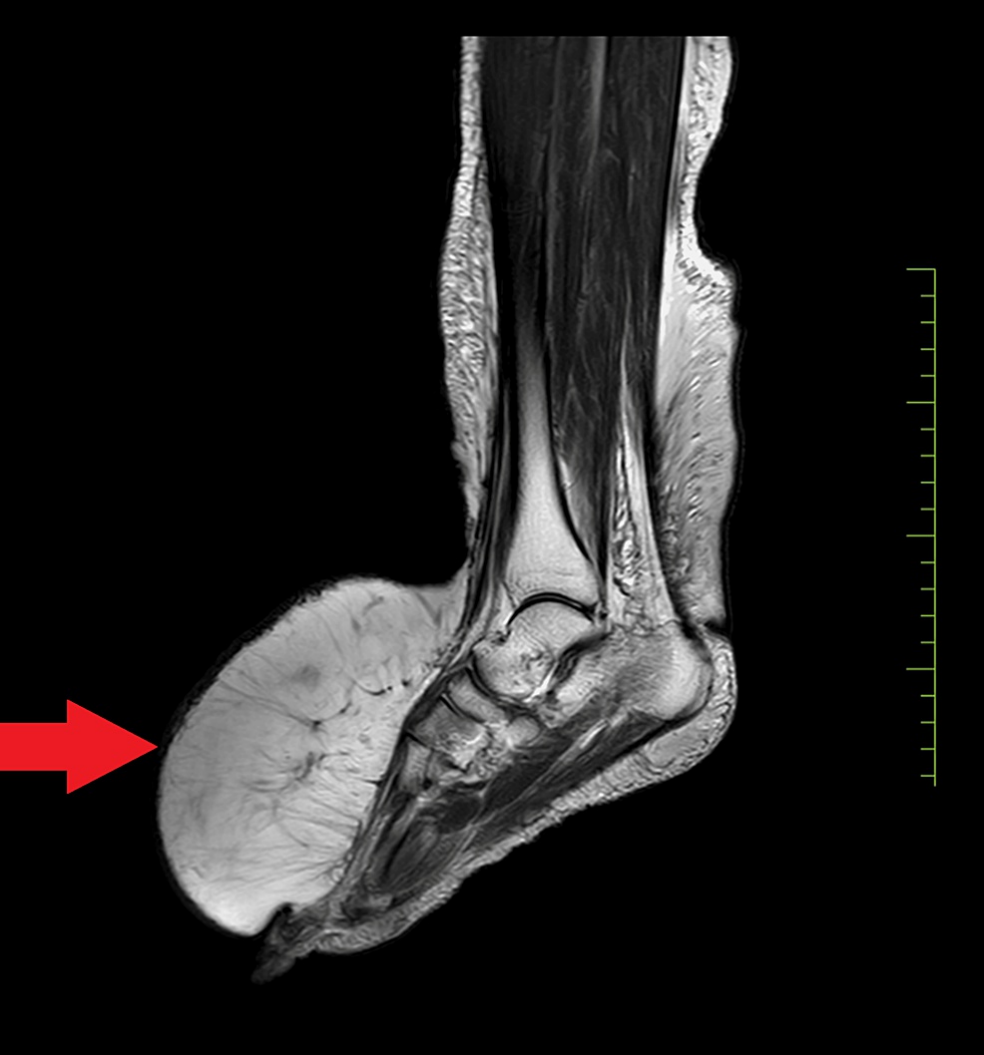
MRI of right leg edema in the dorsum
